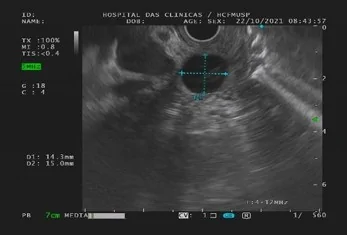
Endoscopic ultrasound confirming the presence of chronic pancreatitis. Source: Personal archive.
